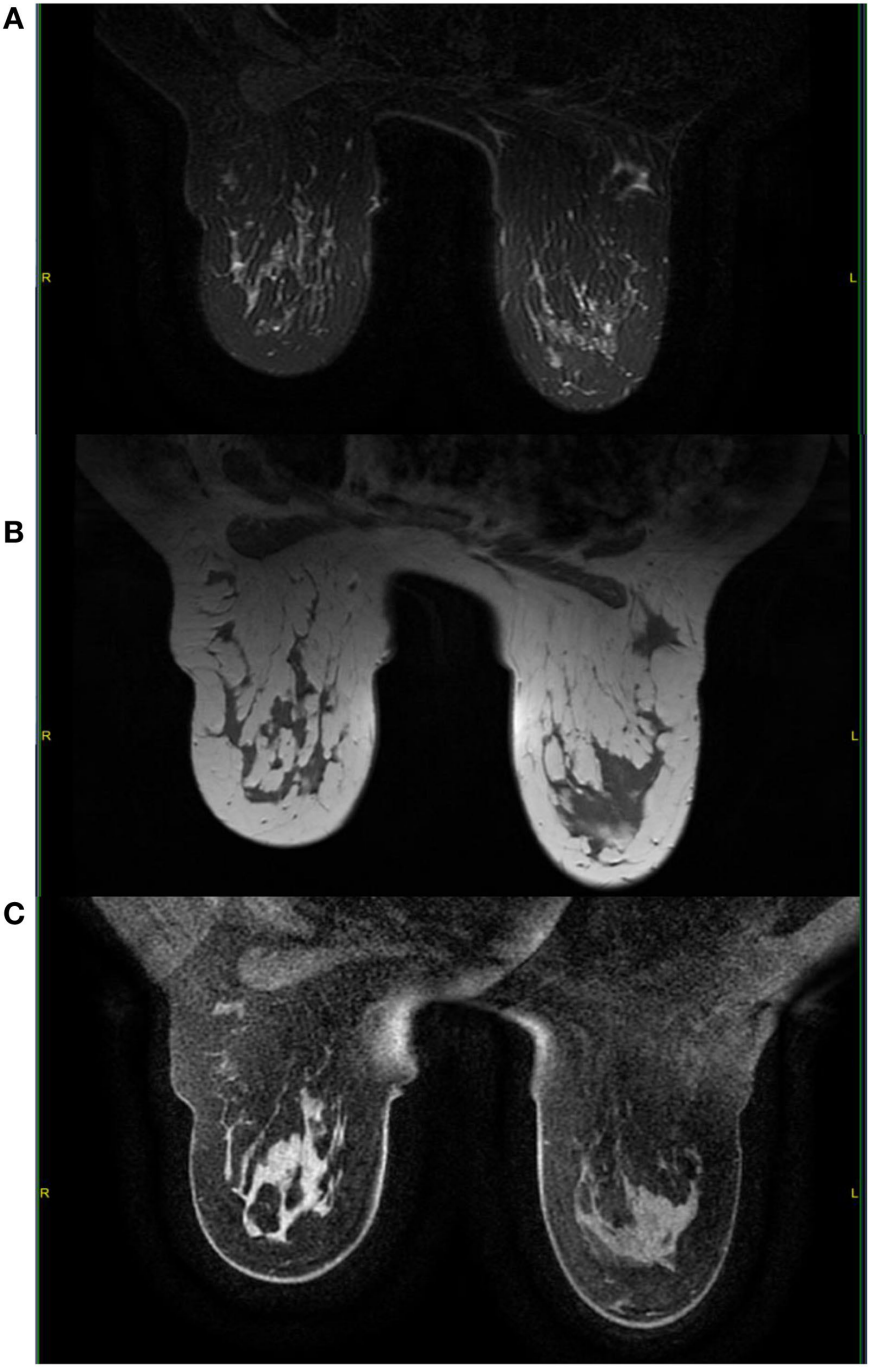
Here, we observe a complete response of the known palpable lesion in the left breast with absence of contrast enhancement (arrow). T2-weighted images with fat saturation (A); T1-weighted images (B); gadolinium-enhanced T1-weighted images with fat saturation (C).
